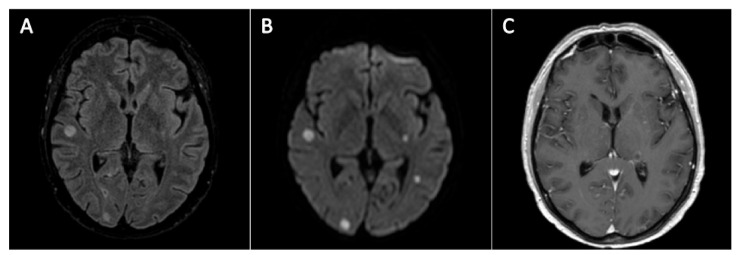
Magnetic resonance imaging of the brain for case 1. Findings were consistent with septic emboli. Pictures (A) and (B) show multifocal superficial and deep bilateral rounded lesions, some of which show nodular or ring-like enhancement after contrast injection (left thalamus in picture (C)). Lesions were localized at both sub- and sovra-tentorial level, with a miliary distribution, the largest ones involving the cerebellum and the brainstem with a maximum diameter of about 10 mm. In addition, phlogistic mucosal thickening of paranasal cavities was documented (not shown). (A): FLAIR sequence; (B): DWI sequence; (C): T1-post Gadolinium sequence.
